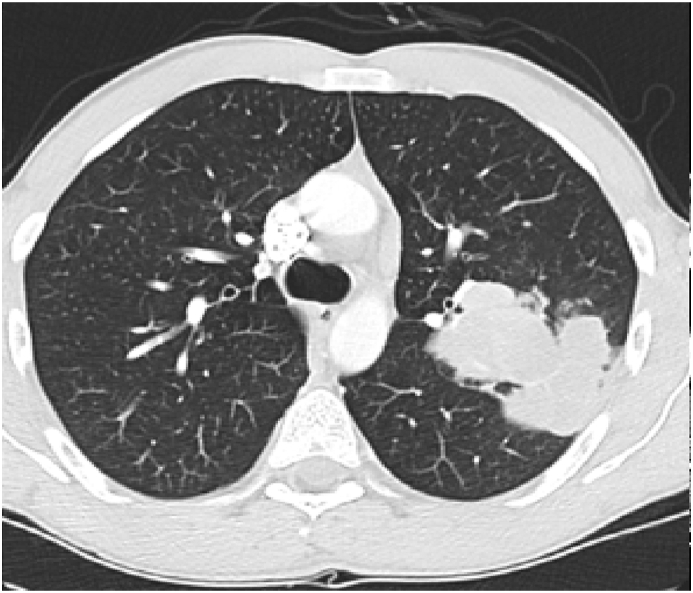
CT chest, April 2020: lung and mediastinal windows respectively, showing a lobulated mass-like lesion.
